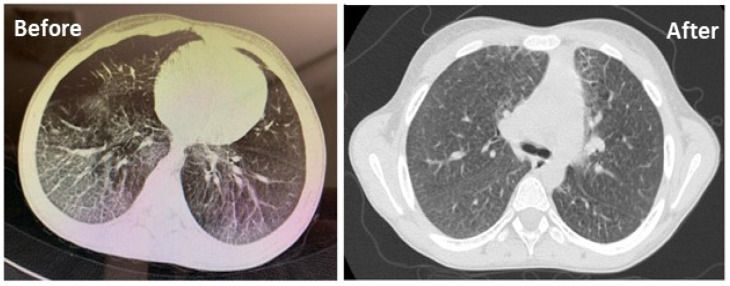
HRCT scan of the chest showing ground–glass changes in both lung fields before and after 3 months of treatment with trehalose in a patient.
